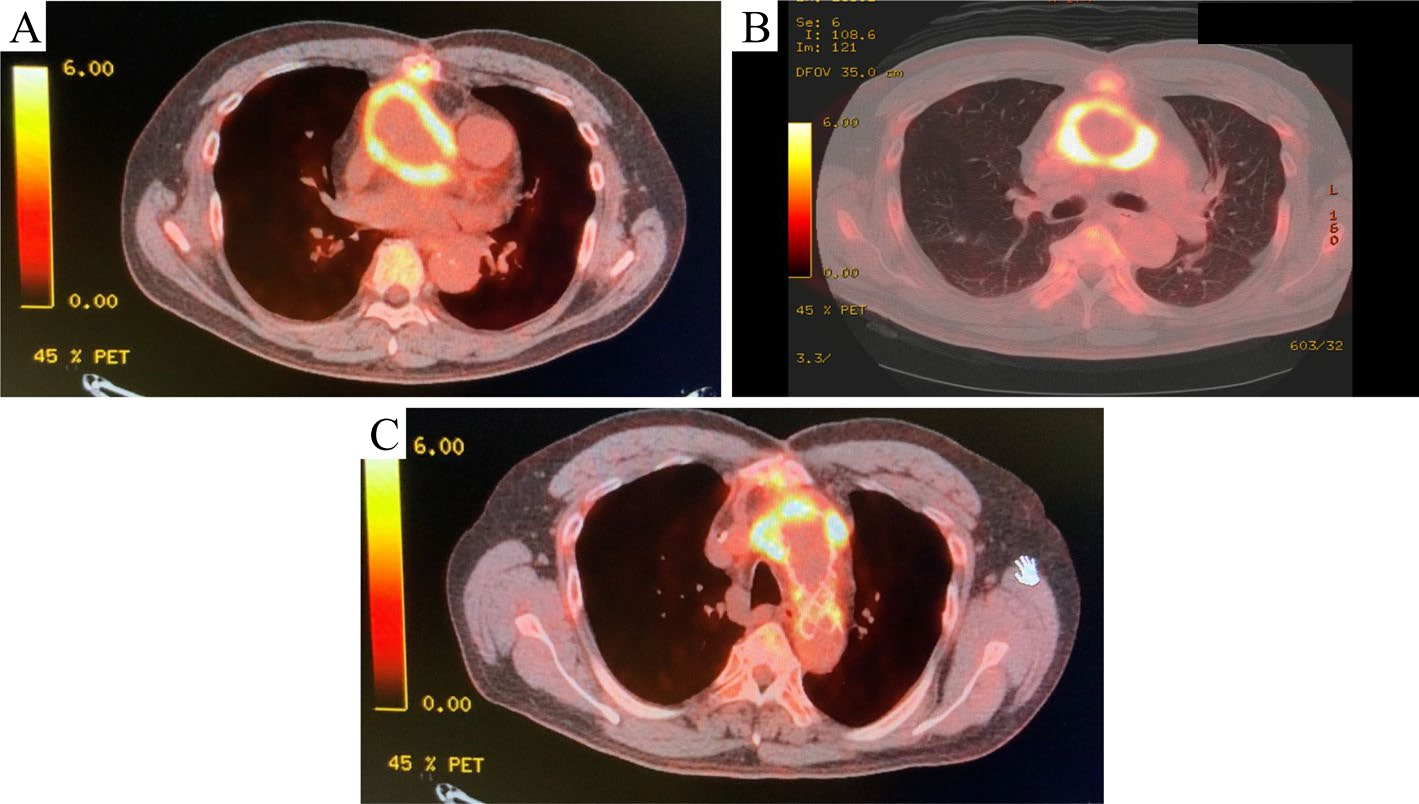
18-Fluorodeoxyglucose positron emission tomography (PET) showed significantly elevated SUV value. A Level of the aortic root, B ascending aorta, C FET
